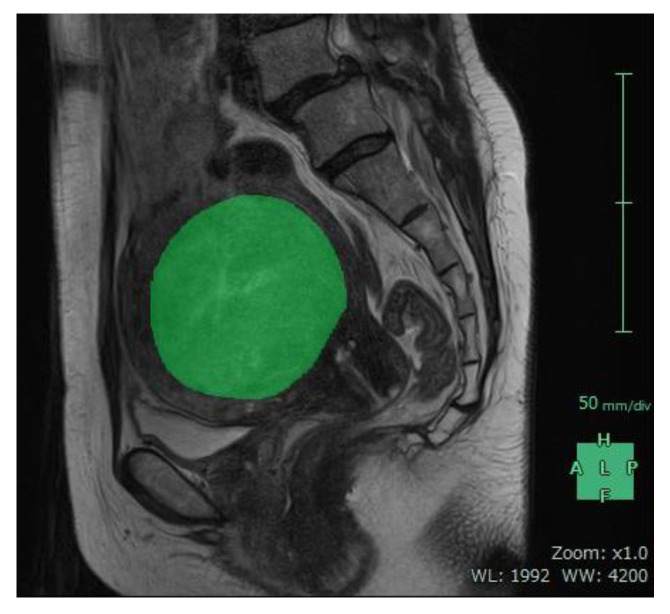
Sagittal MR image from a 48-year-old woman obtained before uterine artery embolization. The margins of the uterine tumor were delineated in sequential sagittal slices similar to those shown in green (mask).
